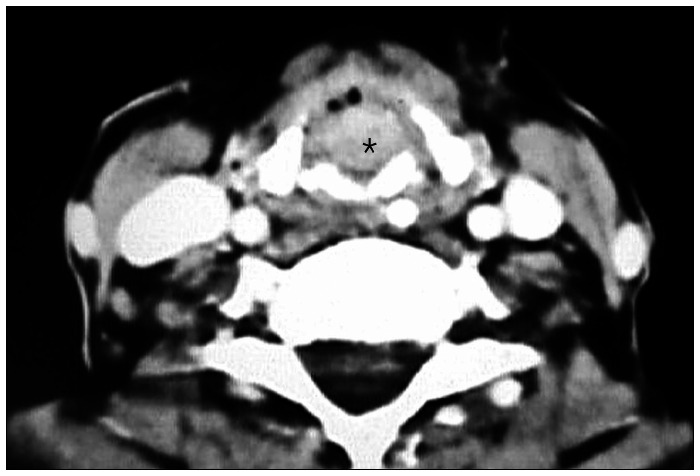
Axial computed tomography scan. Tumor (*) affecting the larynx at the glottis level.
